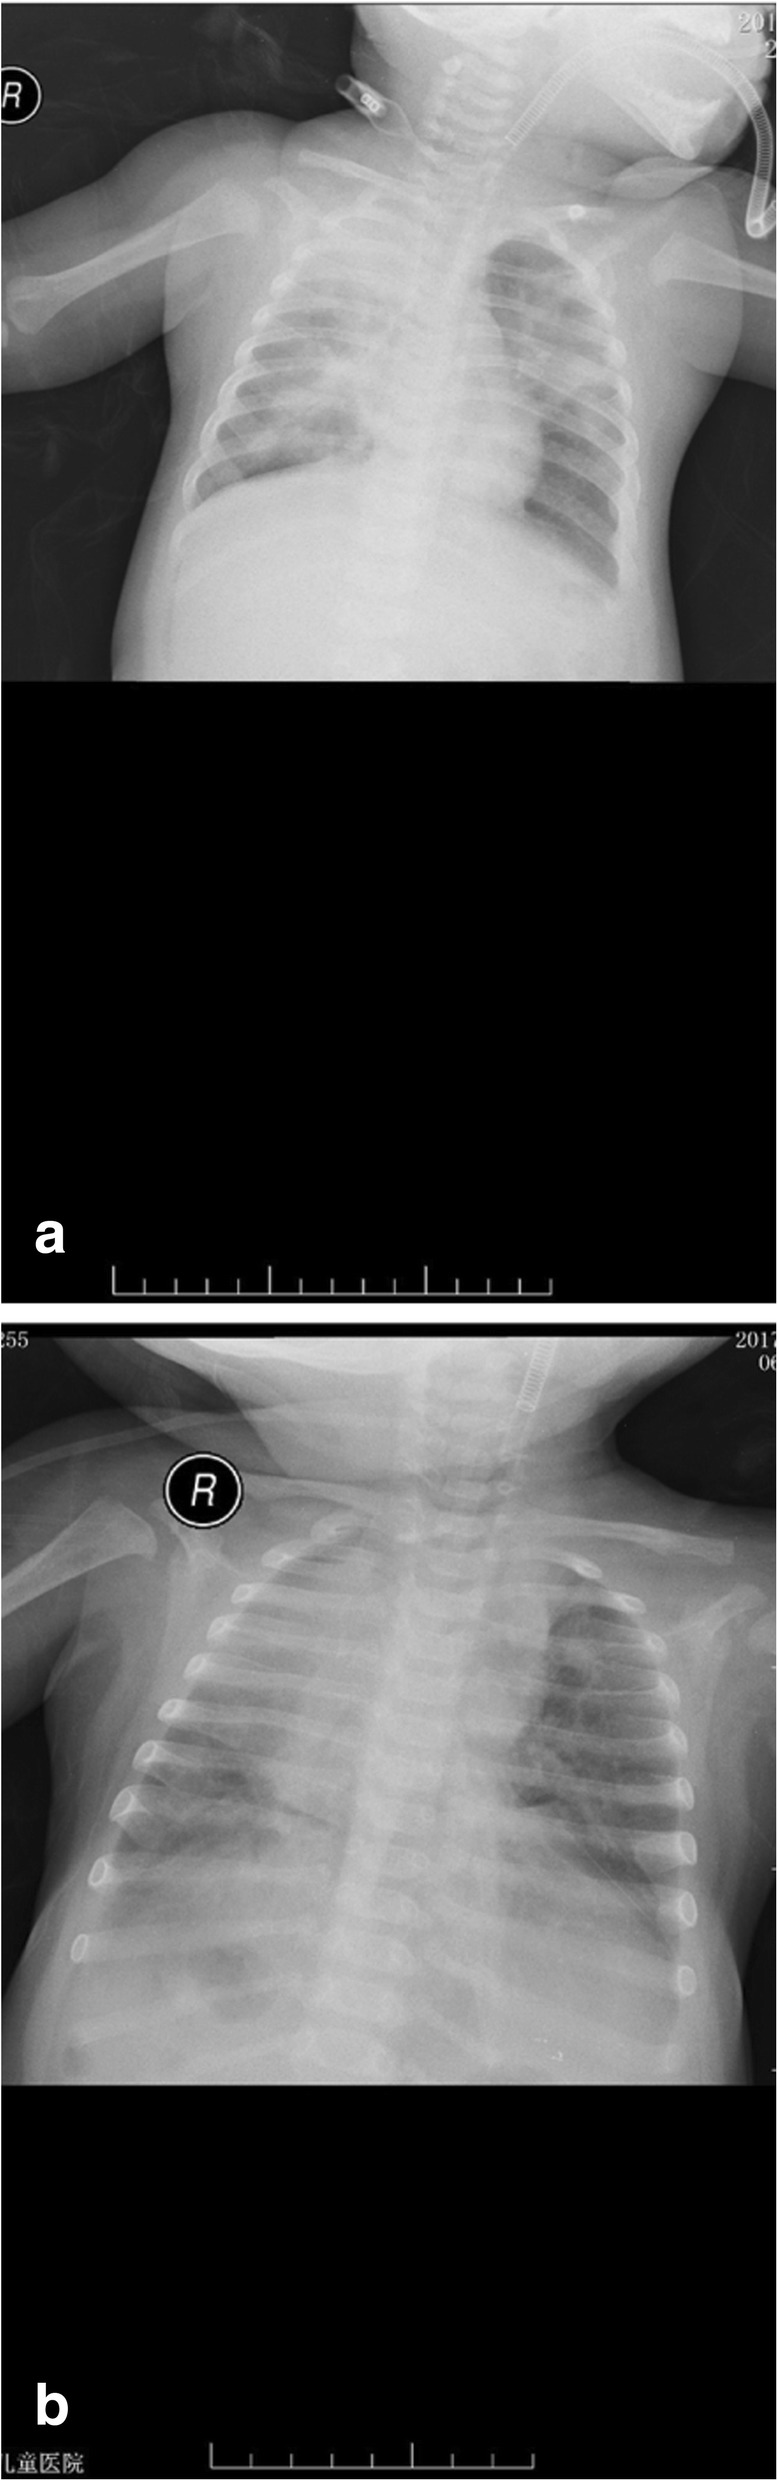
The chest radiograph. (a) on admission, (b) at 48 h after admission
